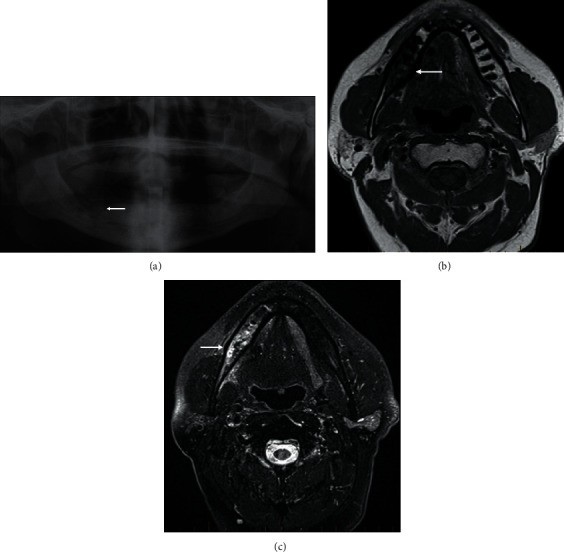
Osteoradionecrosis. Orthopantomogram (OPG, (a)) of a 74-year-old male with a history of T3 oropharyngeal tumour having undergone prior resection and chemoradiotherapy, complicated by osteoradionecrosis. There is ill-defined lytic lucency eroding the right mandibular body with background coarsened trabeculation and patchy sclerosis. Axial T1 (b) and T2FS (c) of a different patient with a previous history of radiotherapy for skin SCC, presenting with a nonhealing ulcer due to osteoradionecrosis. There are T1 hypointensity and fluid-hyperintensity in the body of the right mandible ((a) arrow). Mild surrounding soft tissue oedema ((b) arrow).
